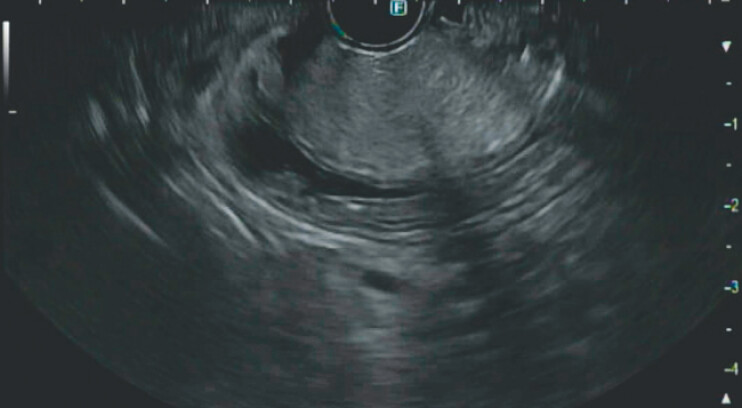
Endoscopic ultrasound showing a duodenal lipoma.
